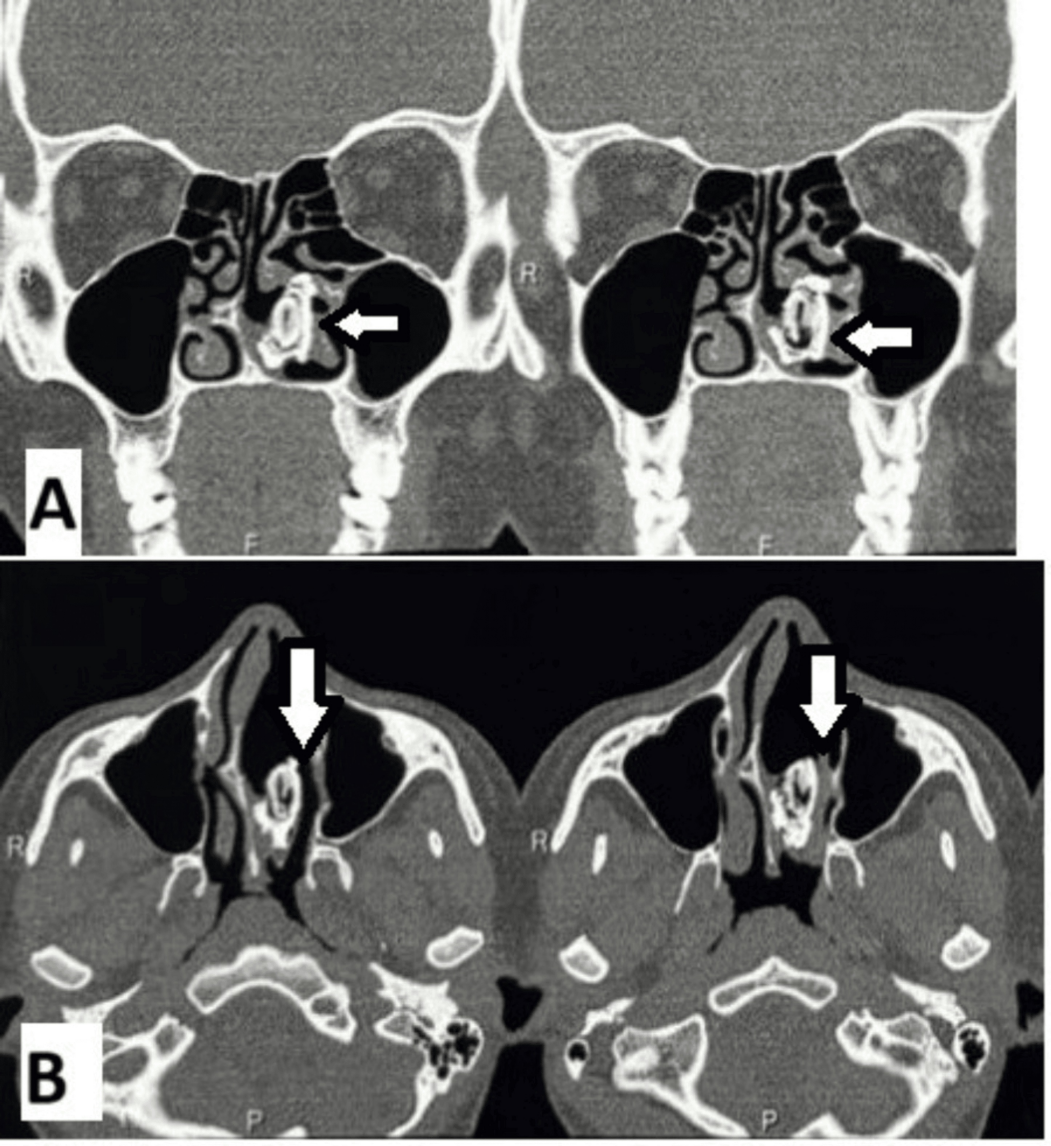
Computed Tomography Scans of the Paranasal Sinus (A) Coronal images of the paranasal sinuses show a radio-dense lesion (white arrow) abutting the inferior and middle turbinates. (B) Axial images of the paranasal sinuses reveal a radio-dense lesion (white arrow) measuring 24 x 12 x 17 mm within the left nasal cavity, laterally indenting the left inferior and middle turbinates and medially abutting the nasal septum. A septal deviation towards the right is also noted.
